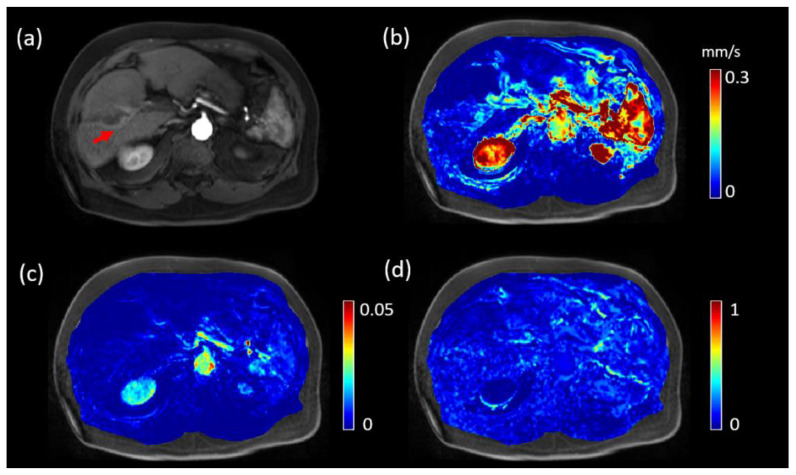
DCE MRI, QTM velocity, $K^{trans}$ and $V_{e}$ maps of HCC of a 74-year-old patient with lung shunting fraction 9.3%. (a) post-Gd T1 weighted image showing the tumor (red arrow). (b) QTM |*u*| map, (c) $K^{trans}$ map, and (d) $V_{e}$ map.
